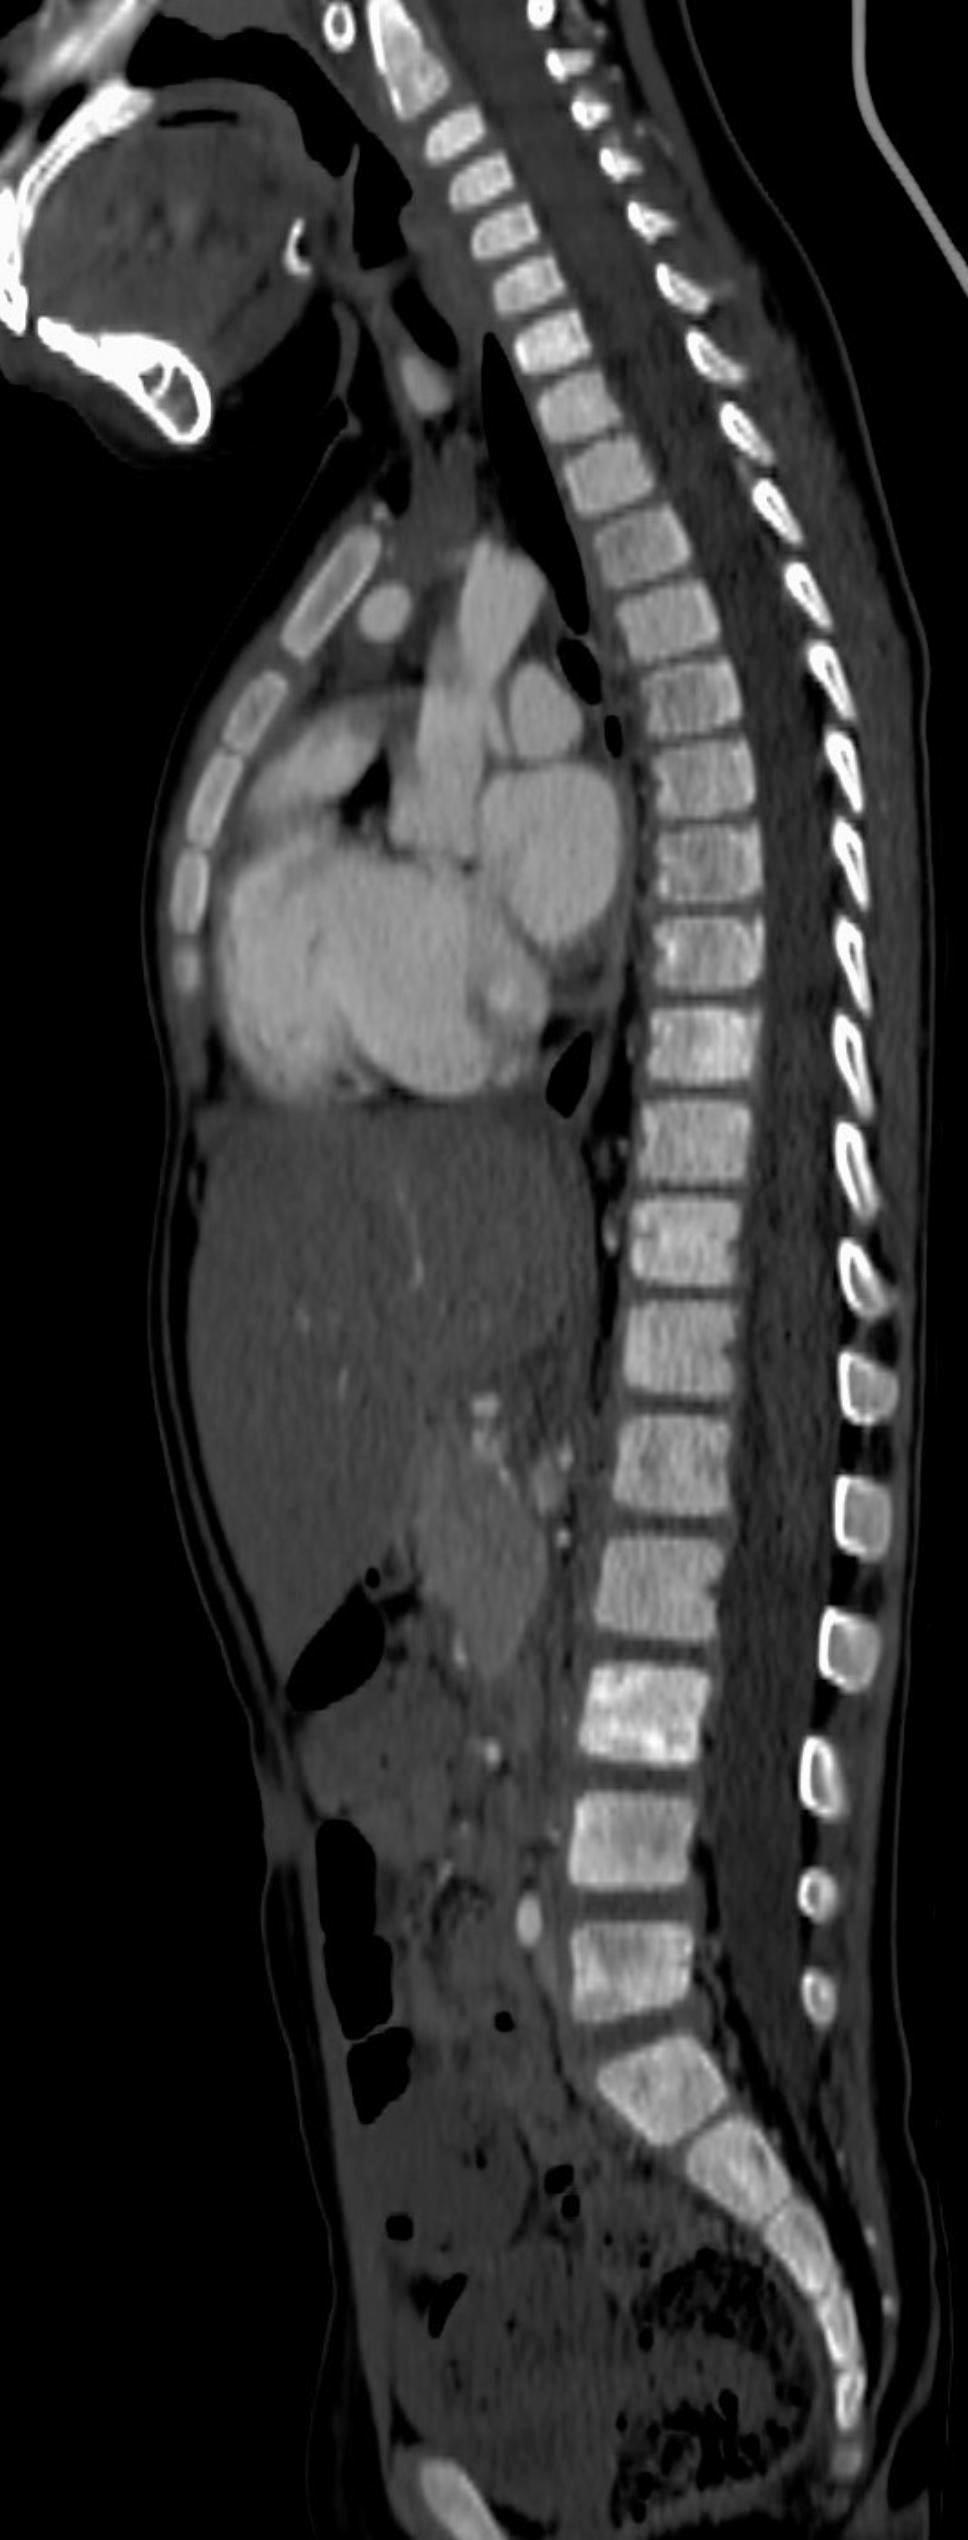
CT scan shows extensive inhomogeneity of the vertebral metamers with hyperdense and osteolytic areas
